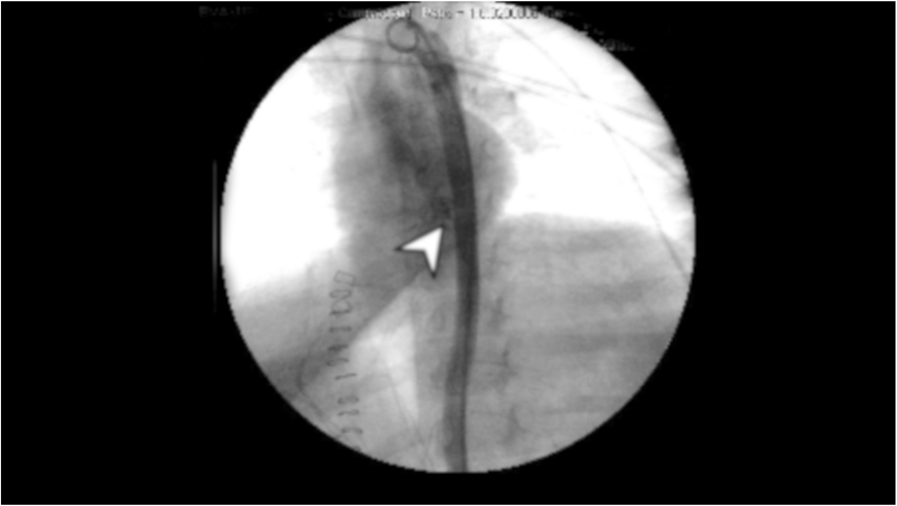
Extravasation of contrast from aorta to the esophagus as shown by the arrowhead (X-ray view)
